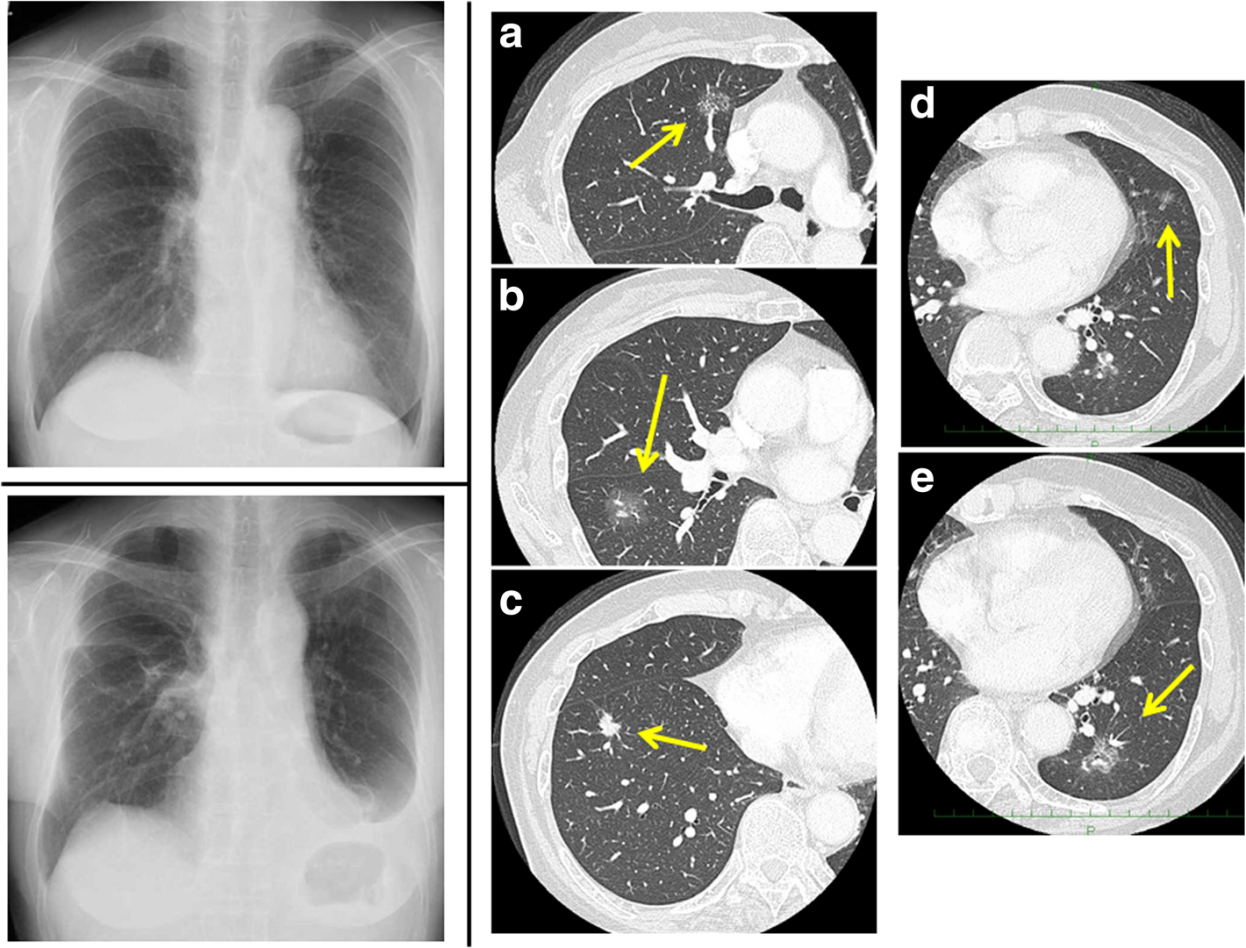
(Left, upper) Chest roentgenogram at presentation. (Right) Preoperative computed tomography (CT) showing bilateral lung nodules as follows: a part-solid ground-glass nodule (GGN, 23 mm) in the anterior segment (S3) of the right upper lobe, b pure GGN (24 mm) in the superior segment (S6) of the right lower lobe, c solid nodule (13 mm) in the anterior basal segment (S8) of the right lower lobe, d pure GGN (8 mm) in the lingular segment of the left upper lobe, and e part-solid GGN (35 mm) in the left lower lobe. (Left lower) Chest roentgenogram after two-staged bilateral operations
